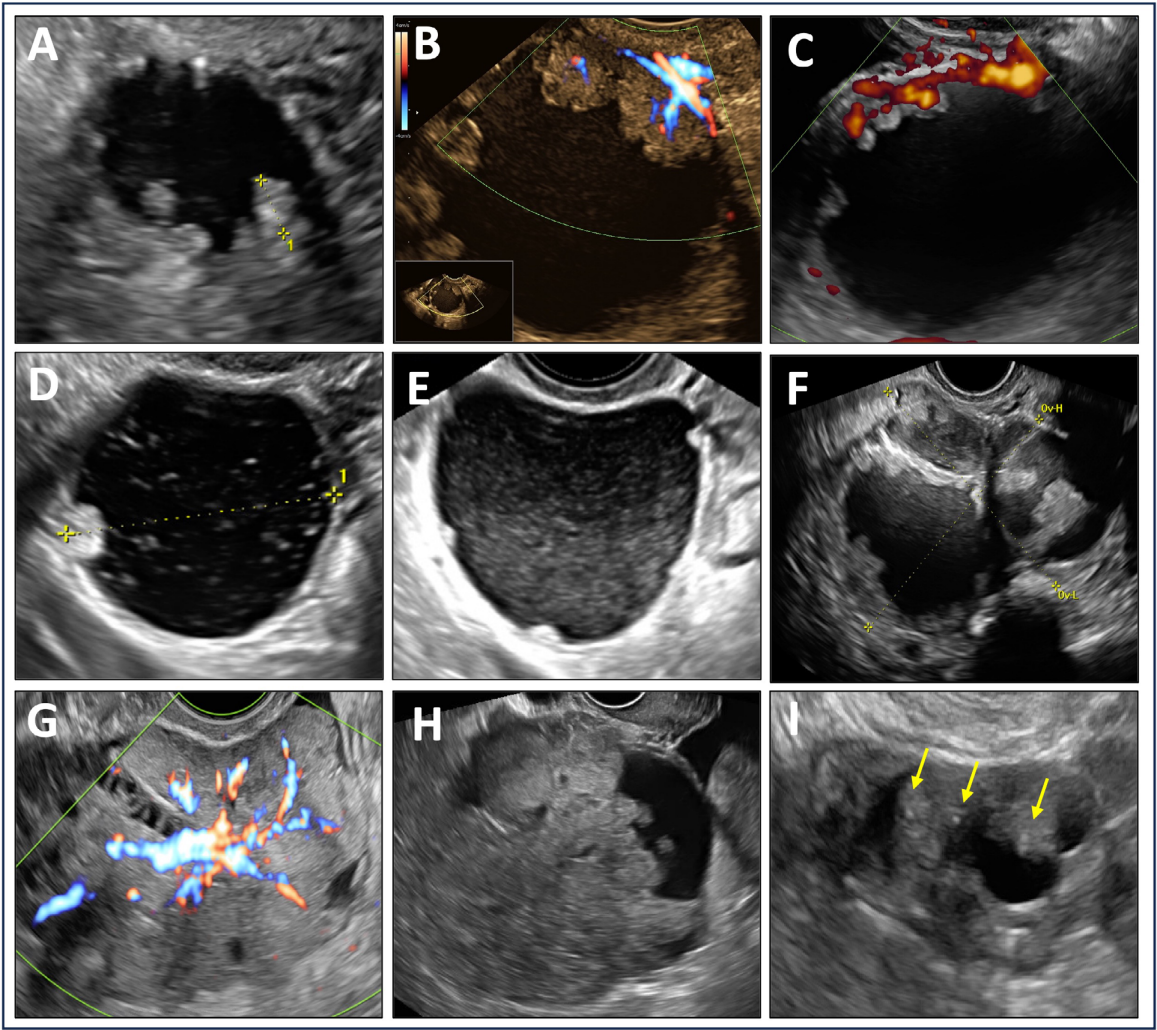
Serous ovarian tumors. Serous borderline ovarian tumors usually appear on ultrasonography Doppler imaging as cystic lesions with numerous vascularized papillary projections (A–F). Rarely, however, they may show an exophytic growth pattern with predominantly solid tumors that exhibit rich hierarchical vascular branching (i.e., a fireworks sign; G). Serous low‐grade ovarian cancer (H, I) often presents as irregular solid lesions with exophytic growth (H) or cystic lesions with endophytic papillary projections (I). Hyperechoic foci representing small calcifications may also be present (yellow arrows in I).
